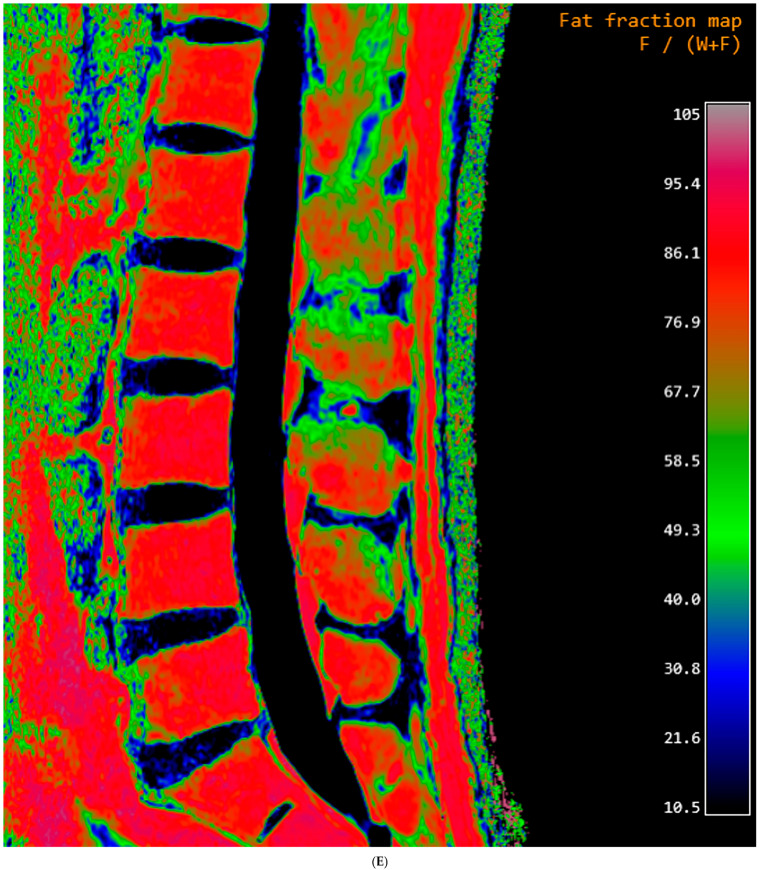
Lumbar spine MRI of a 59-year-old male. (A) Fat fraction (FF) measurement on gradient-echo (GRE)-based chemical-shift-encoded magnetic resonance imaging (CSE-MRI). The FF_GRE_ in this patient was 66.91%, representing the median value of the L1–L4 vertebral bodies. (B) FF measurement on spin-echo (SE)-based CSE-MRI. The FF_SE_ was 85.84%. The numbers in the figure indicate the ROI numbering in the EXPRESS program. (C) Measurement of the vertebral bone quality (VBQ) score was calculated by dividing the median value of T1-weighted signal of L1–L4 vertebral bodies by the cerebrospinal fluid (CSF) signal at the L3 level. The VBQ score for this patient was 2.63. (D,E) FF color maps were created through GRE- and SE-based CSE-MRI.
